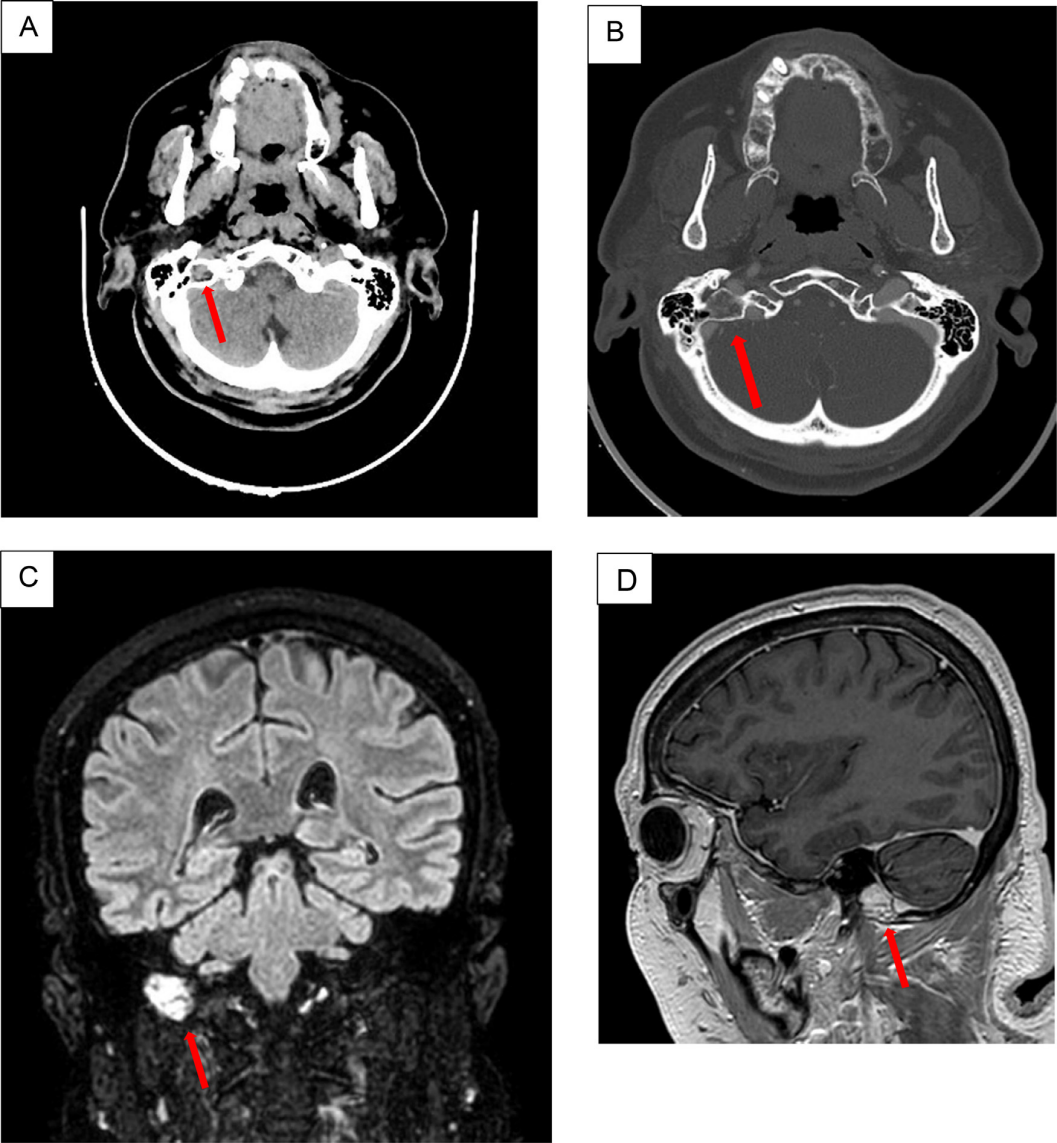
(A) *Axial view*: CT scan shows the presence of oval formation in the medial side of the right mastoid, at occiputmastoid junction, side leading to cancellous bone swelling and bone cortical thinning with parenchymatous-like density. (B) Axial view: CT scan – bone window, slice 1.25 mm: note the trabeculation of the bone thinned and remodeled by the presence of the angioma. (C) *Coronal view*: MR Flair shows a hyperintense signal with a “bunch of grapes” appearance in right temporal bone. (D) *Sagittal view*: T1-weighted gadolinium enhanced MR shows expansive.
